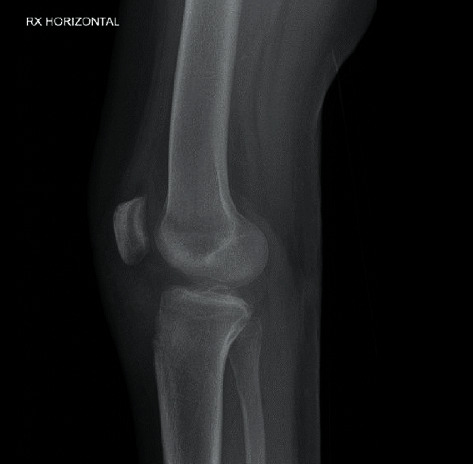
Lateral radiographic view of the knee at a 9-month follow-up. Radiographs demonstrated a normal patellar height with a Caton-Deschamps index measured at 0.7; however, we noted an anterior static tibial translation of 9 mm.
